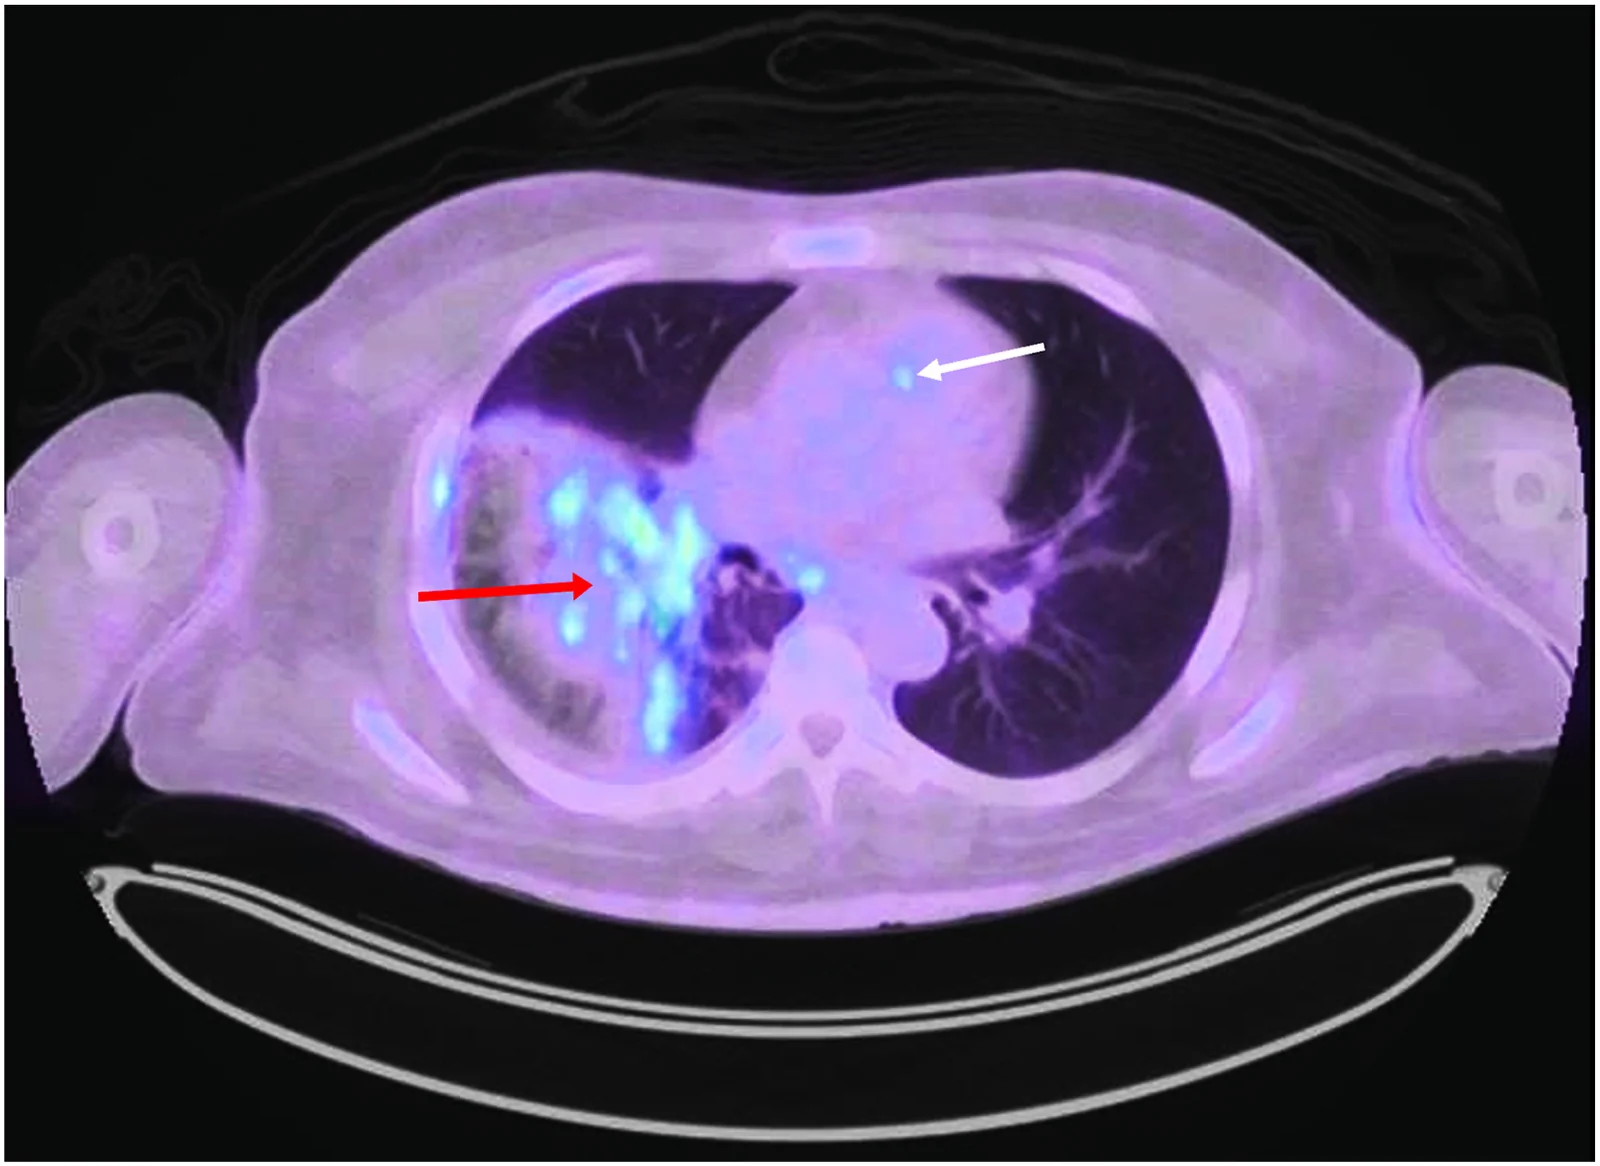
Fluorodeoxyglucose-PET/CT (axial fusion image) demonstrates focal hypermetabolic uptake at the PV *(white arrow)* and increased metabolic activity within the right lung consolidation *(red arrow)*, consistent with septic emboli.
